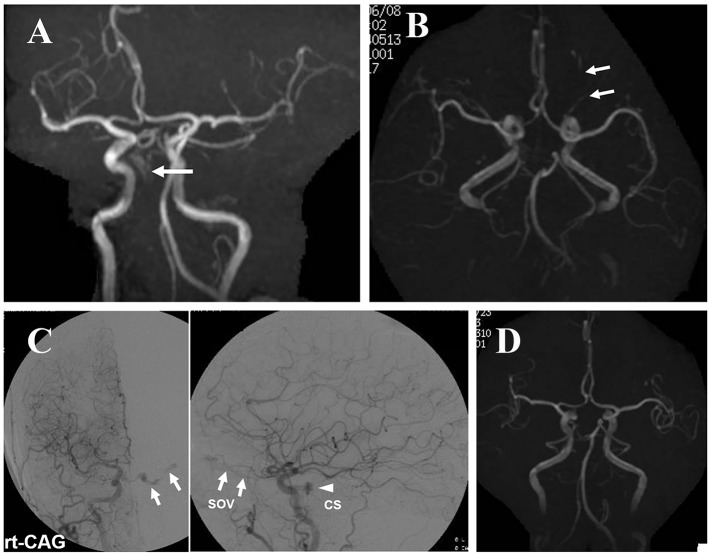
Case 2: (A) MRA performed 1 month after the initial headache demonstrating an abnormal intensity in the right CS (arrow). Reflux to and dilatation of the SOV are not apparent. (B) MRI after the patient had become aware of double vision, again showing blood reflux to and dilatation of the left SOV (arrows). (C) Angiography showing arteriovenous shunt to the right CS and reflux to the left SOV. No blood reflux to a cortical vein is observed. (D) MRA after manual compression of the carotid arteries showing the disappearance of blood reflux to the left SOV.
